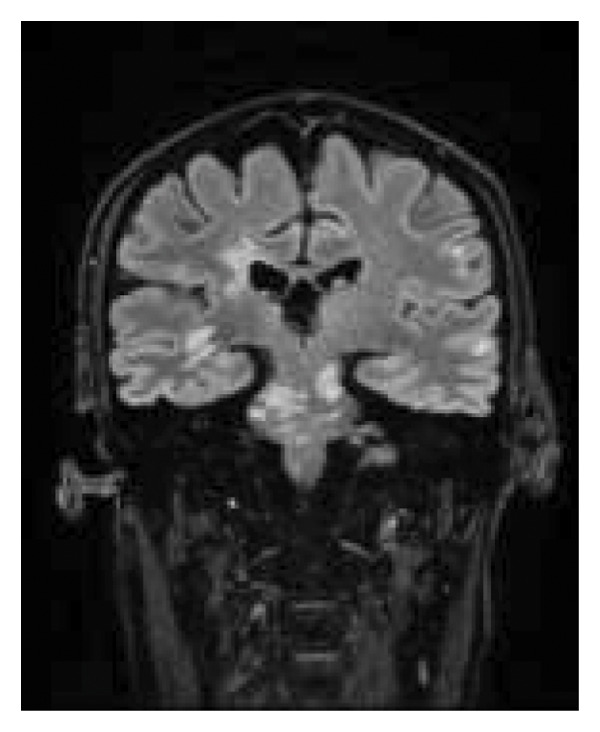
Coronal brain MRI, FLAIR sequence. Radiological worsening following corticosteroid tapering.
